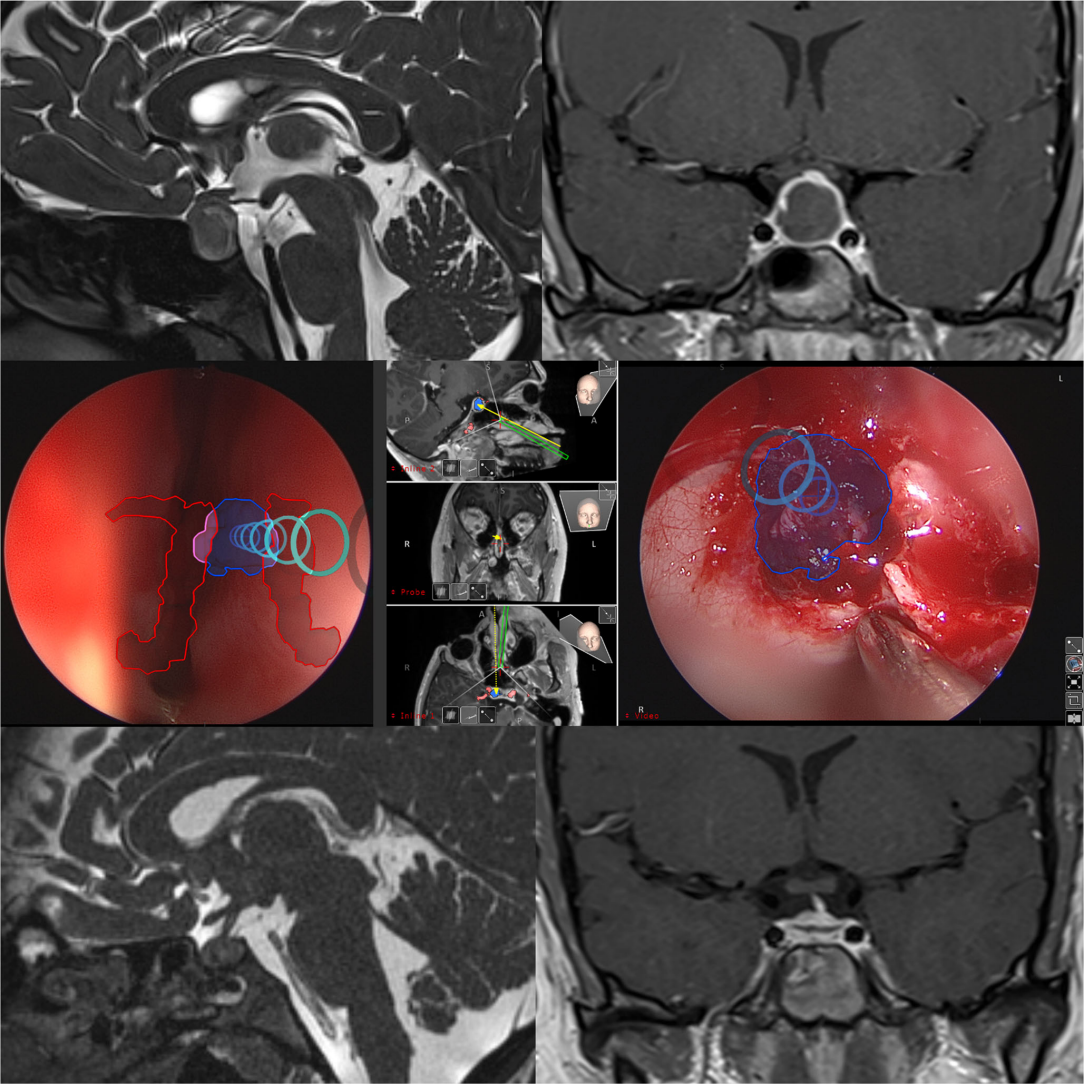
Case example of a patient with a Rathke cleft cyst: upper row: preoperative MRI (T2 sagittal and T1 post-gadolinium coronal sequence). Middle row: intraoperative endoscopic view with AR information indicating the trajectory (multiple rings), the target (blue contour) and the carotid arteries. Lower row: postoperative MRI (T2 sagittal and T1 post-gadolinium coronal sequences)
